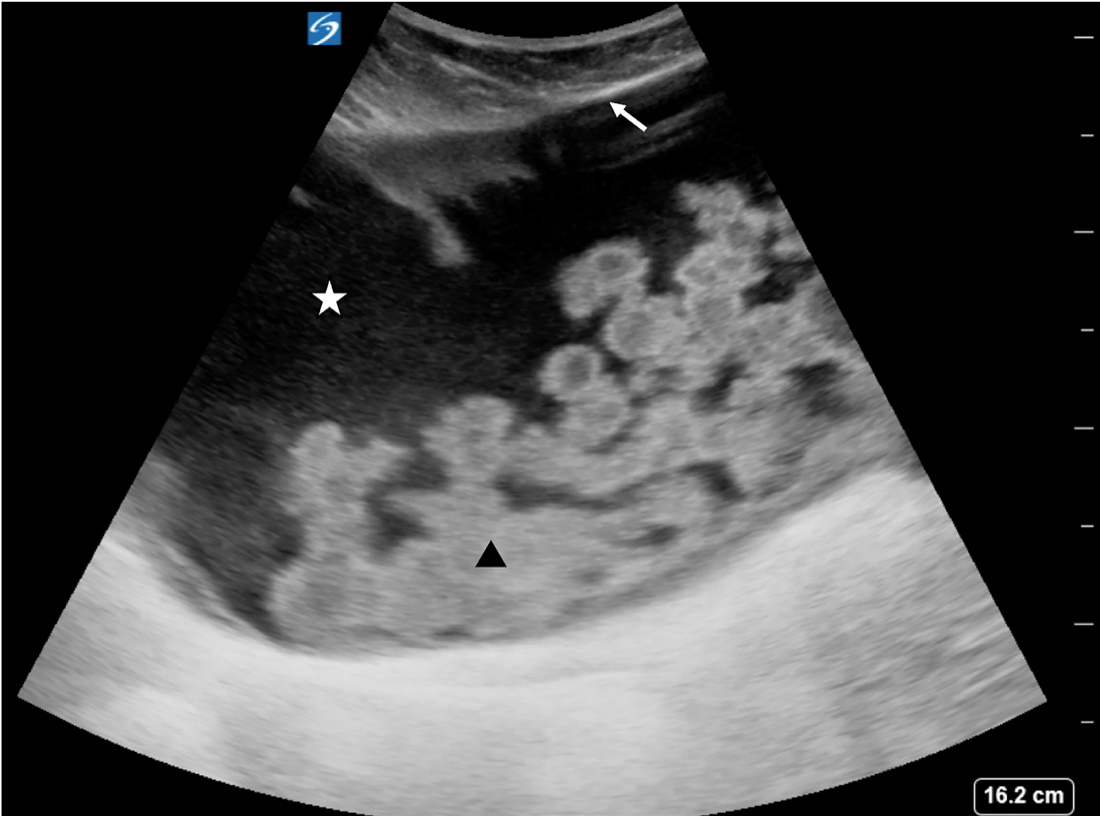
Point-of-care ultrasound performed with a curvilinear probe in the right lower quadrant shows a large, anechoic (black) collection of cerebrospinal fluid (white star) encapsulated by a fibrous layer (white arrow) and containing echogenic debris and hyperechoic (white) septations (black arrowhead).
